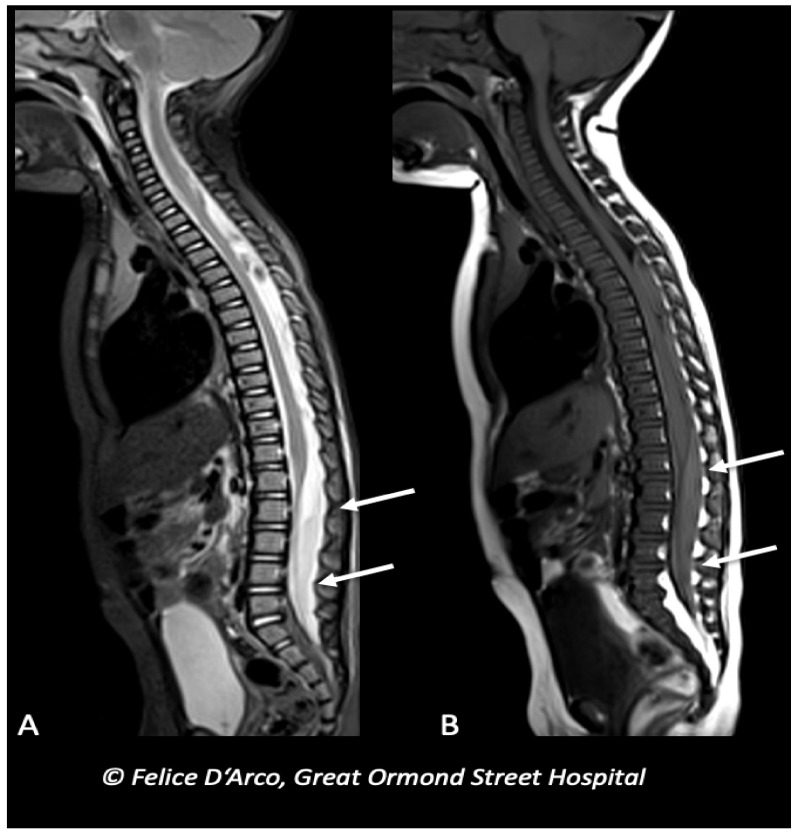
Infant with multiple long bones and ribs fractures (X-ray not shown), and bilateral subdural blood collections at the convexity (brain MRI not shown), subsequently underwent MRI spine examination showing extra-axial mixed-intensity fluid collection indicative of subdural hemorrhages at the level of lumbar spine (arrows) (A and B, sagittal T1 and T2).
